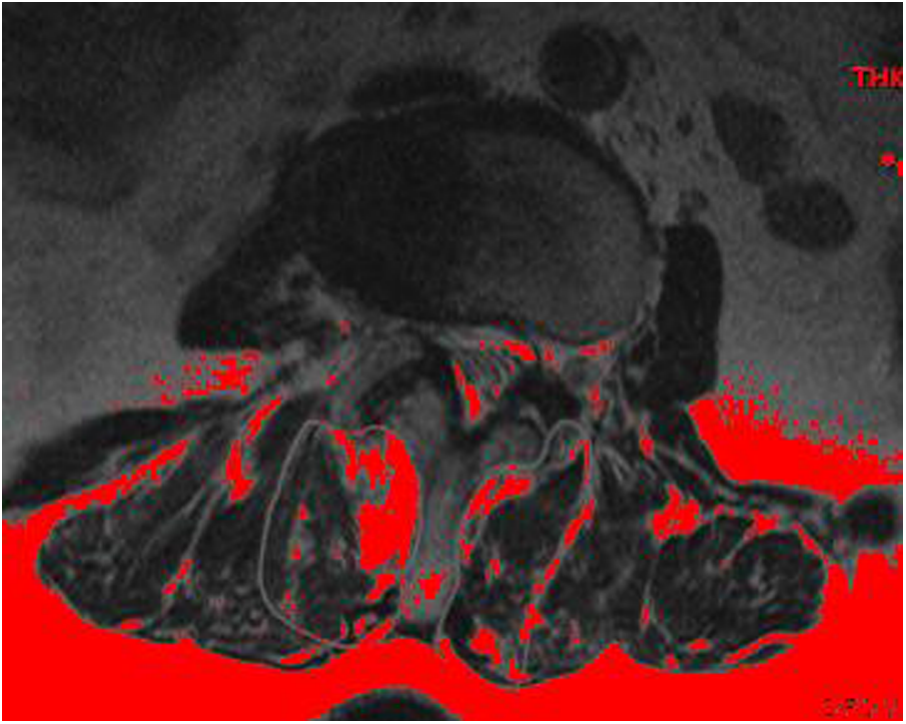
Fat tissue on the MRI is colored in red (a darker background in the red version) using the threshold technique.
